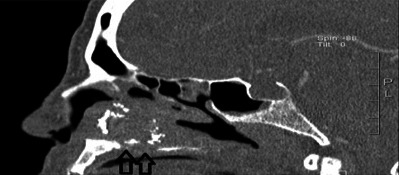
Preoperative sagittal CT imaging of paranasal sinus demonstrating multiple erosions of the hard palate (arrows).
